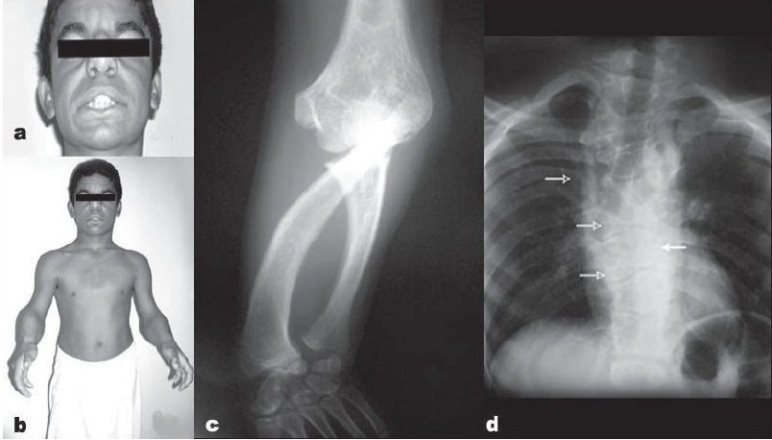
Case 1 (a) Facial features of Robinow syndrome showing broad forehead, hypertelorism, and broad and upturned nose. (b) Profile of patient showing normal upper arm, elbow deformity, and short forearm. (c) Anteroposterior X-ray of forearm with elbow showing dislocated radial head, bowed radius, and hypoplastic ulna (noted bilaterally). (d) Chest X-ray film showing rib anomaly (white arrow), hemivertebrae (white arrows), and block vertebra (bold arrow)
